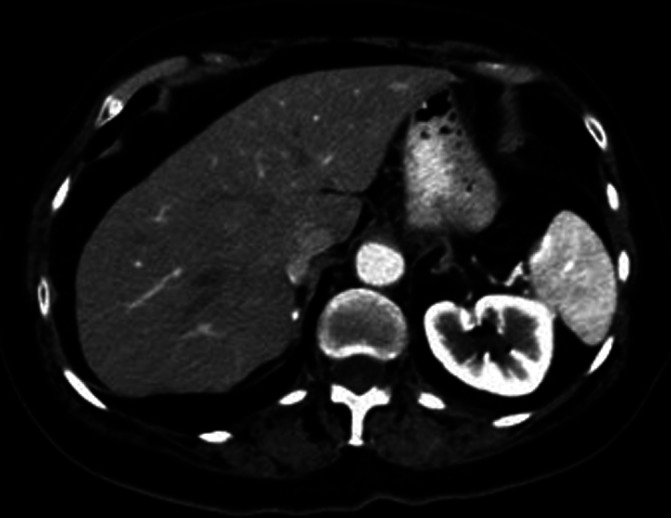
Axial CT SCAN of the abdomen shows no evidence of splenic artery aneurysm. Images acquired February, 2021
